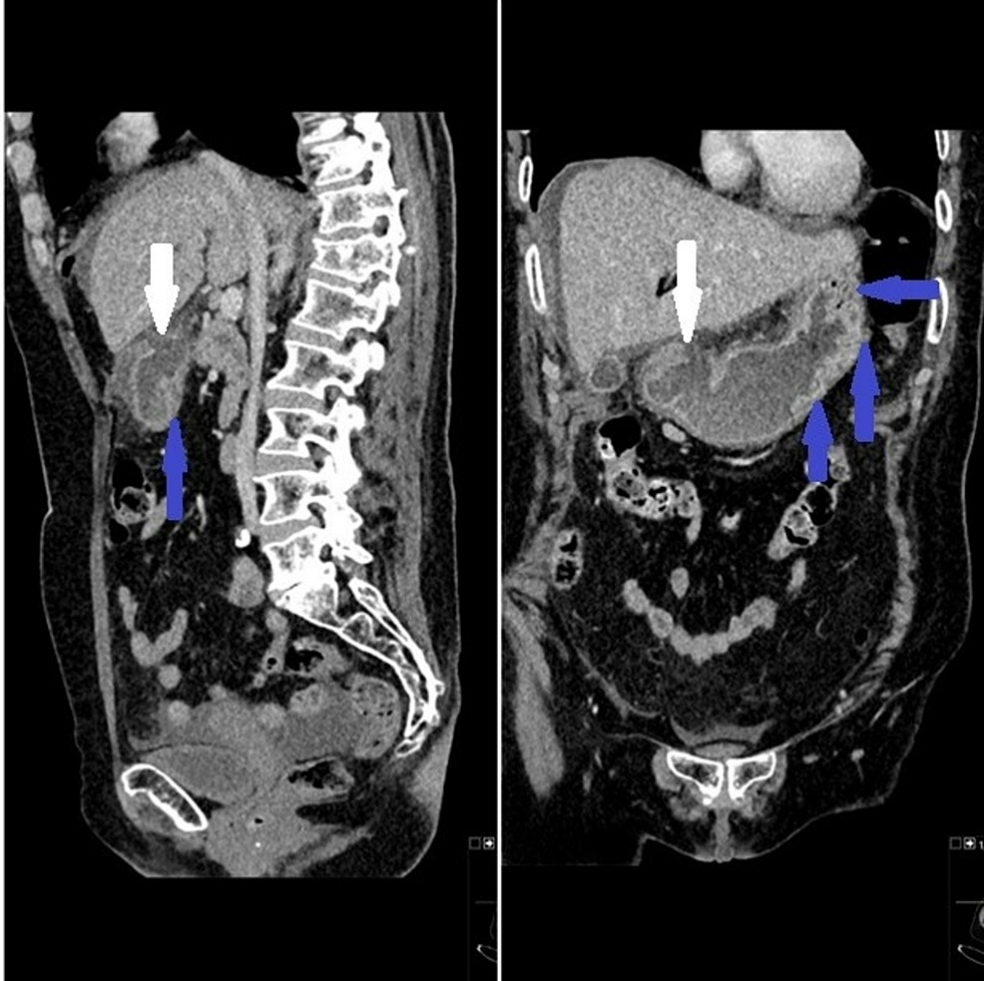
CT coronal and sagittal reformat images showing the defect in the gastric wall (white arrow) and oedema of the gastric wall (blue arrows).
